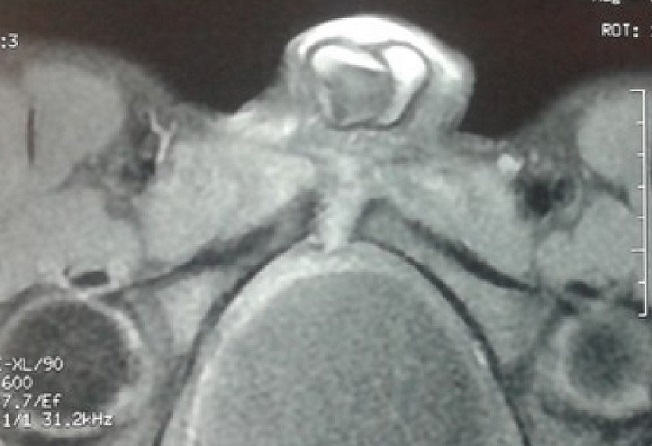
The magnetic resonance imagery (MRI) T1 of the pelvis showed bone oedema of symphysis pubis and abdominal muscles after gadolinium injection
